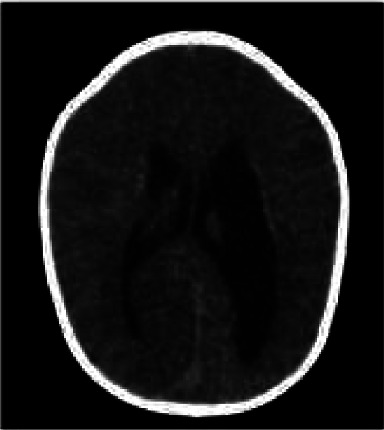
Brain CT, ventricular enlargement, and periventricular calcification.
